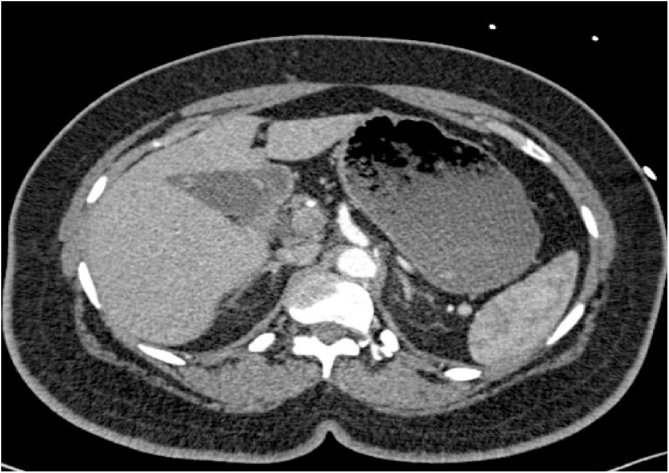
CT scan at the time of original presentation of the patient.
